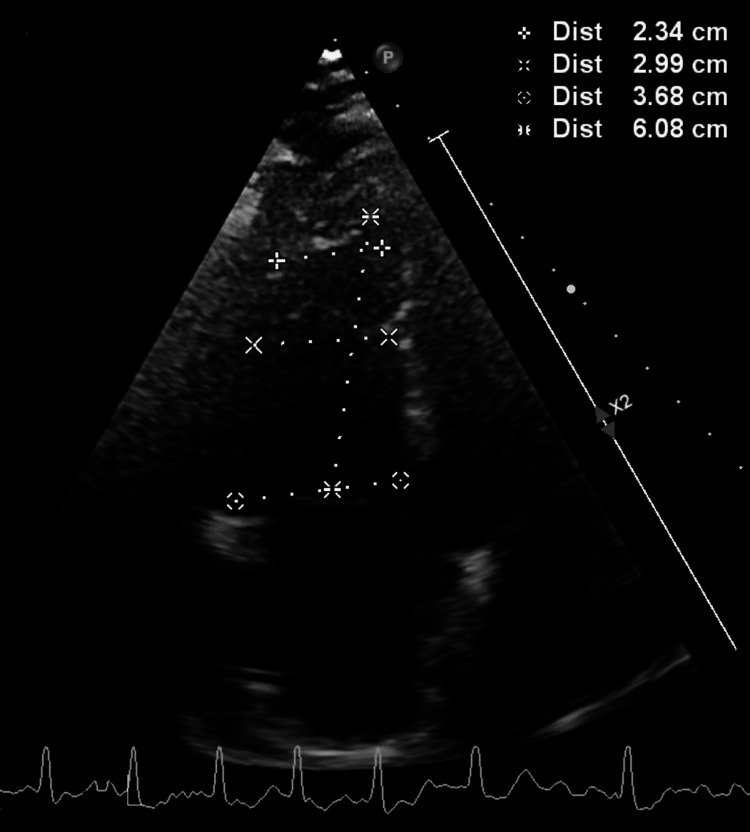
Transthoracic echocardiography (TTE) findings Apical four-chamber view showing a dilated right ventricle
